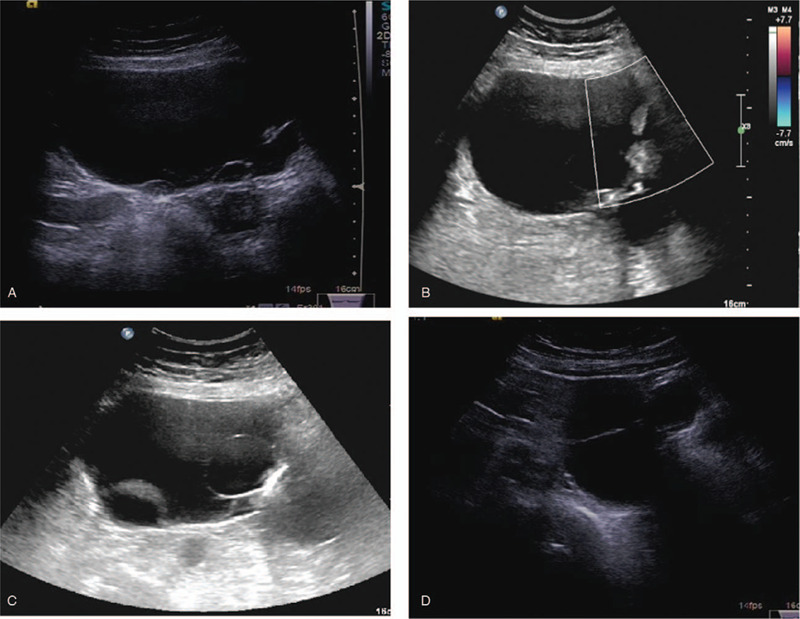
(A) Transabdominal US showed a large multiloculated cystic mass in the right adnexa. (B) No blood flow signals were detected in the papillary projection of the mass by Doppler US. (C) A liner high echo signal with shadows was found within the mass. (D) Another multiloculated mass with thin septa was also seen in the left adnexa. US = ultrasonography.
